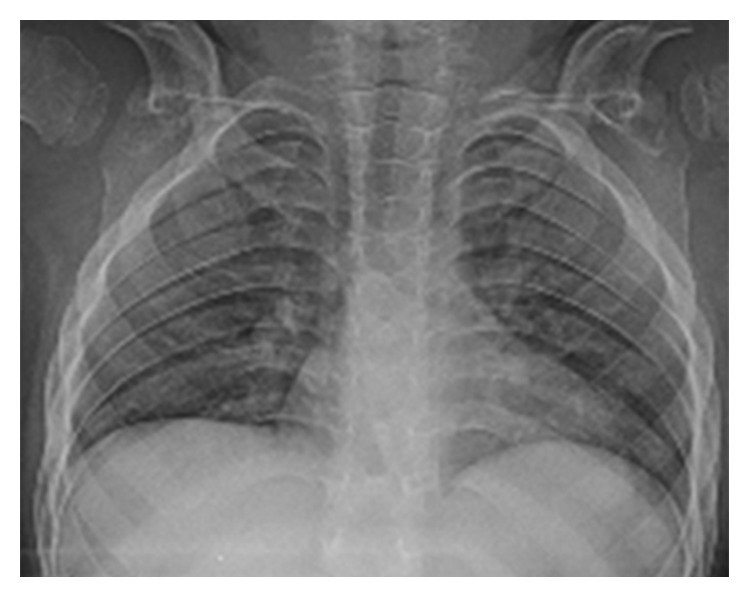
Chest X-ray image revealing oar-like ribs.
